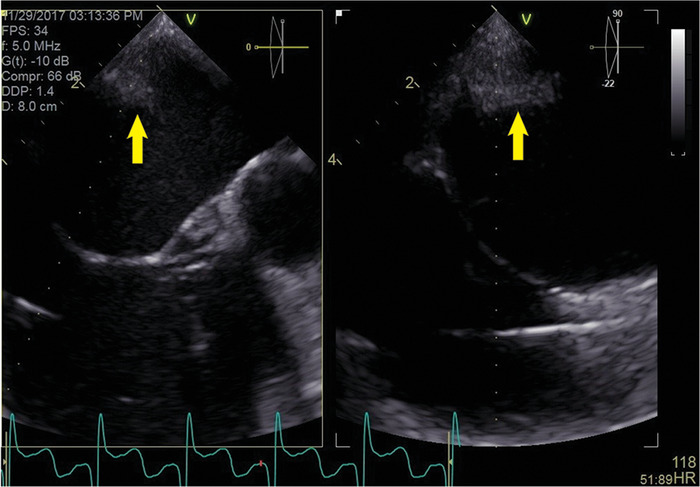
Transesophageal echocardiogram shows 15 × 11 mm posterior wall thrombus (arrows).
